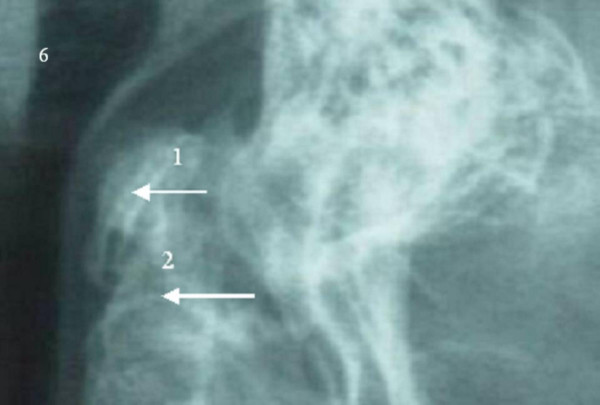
Os odontoideum is seen separated from the odontoid and in relationship to the axis (orthotopic type os, arrow 1). Dynamic x-rays in extension were used to depict the degree of abnormal motion between C1 and C2 (odontoid hypoplasia and axial synchondrosis arrow2). There is anterior instability, with the os odontoideum subluxing forward in relation to the body of C2.
